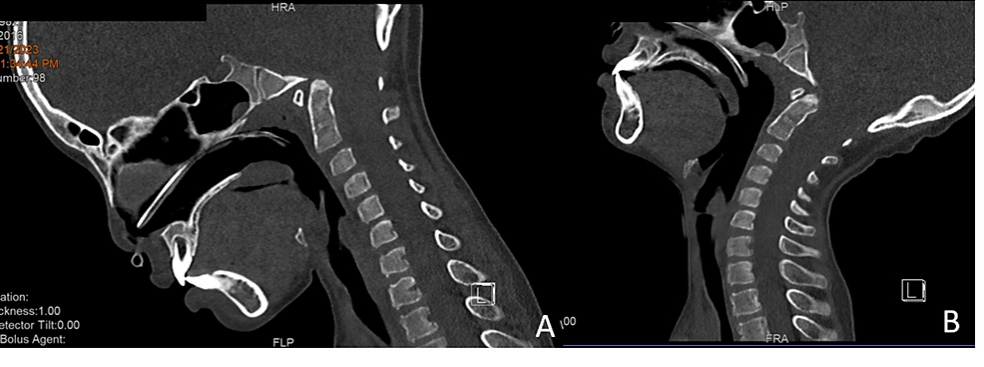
CT cervical in flexion (A) and extension (B) sagittal view on admission shows the widening of the spinal canal on extension CT: computed tomography
